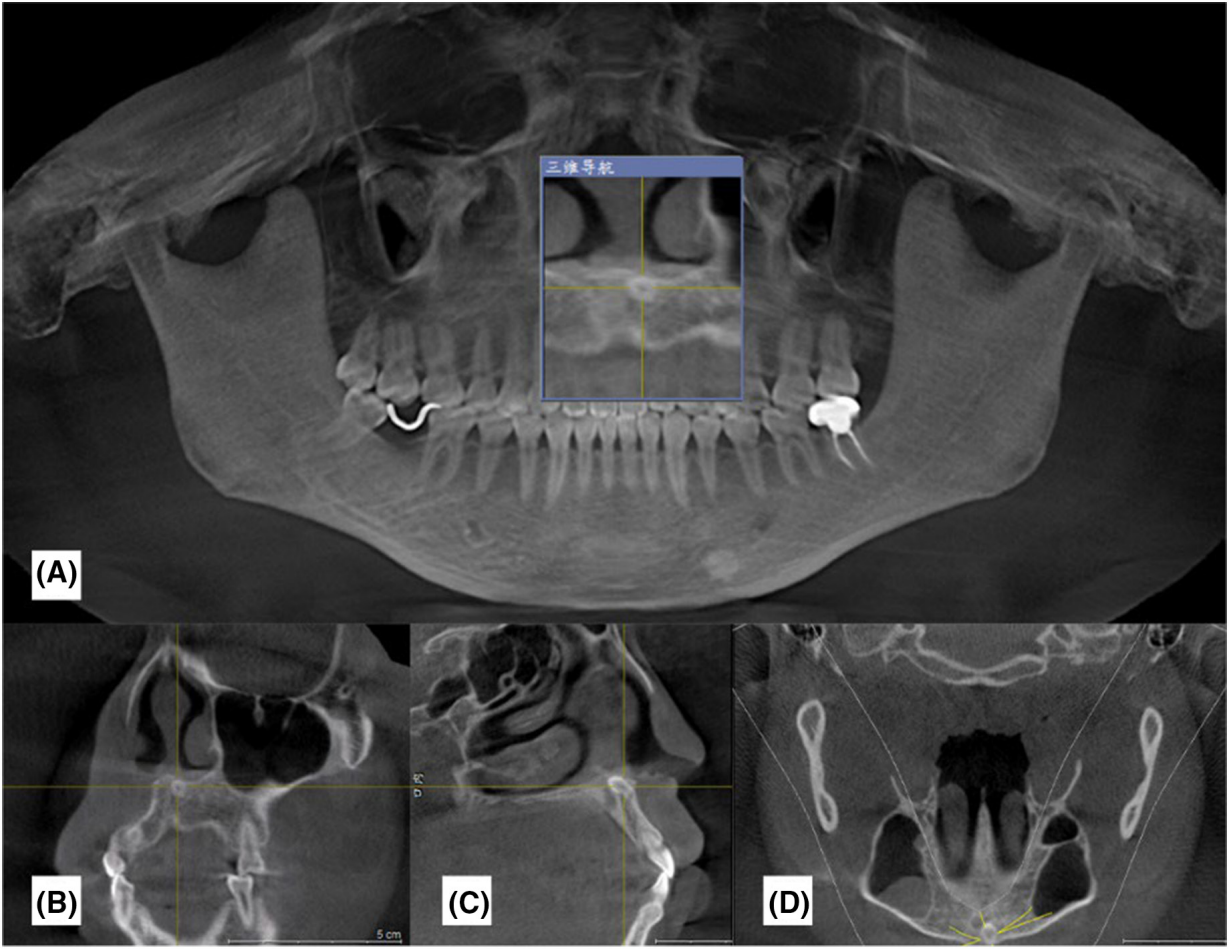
Preoperative CBCT images of the patient. (A)Preoperative pantomography; (B) cross section; (C) longitudinal section; (D) horizontal position.
